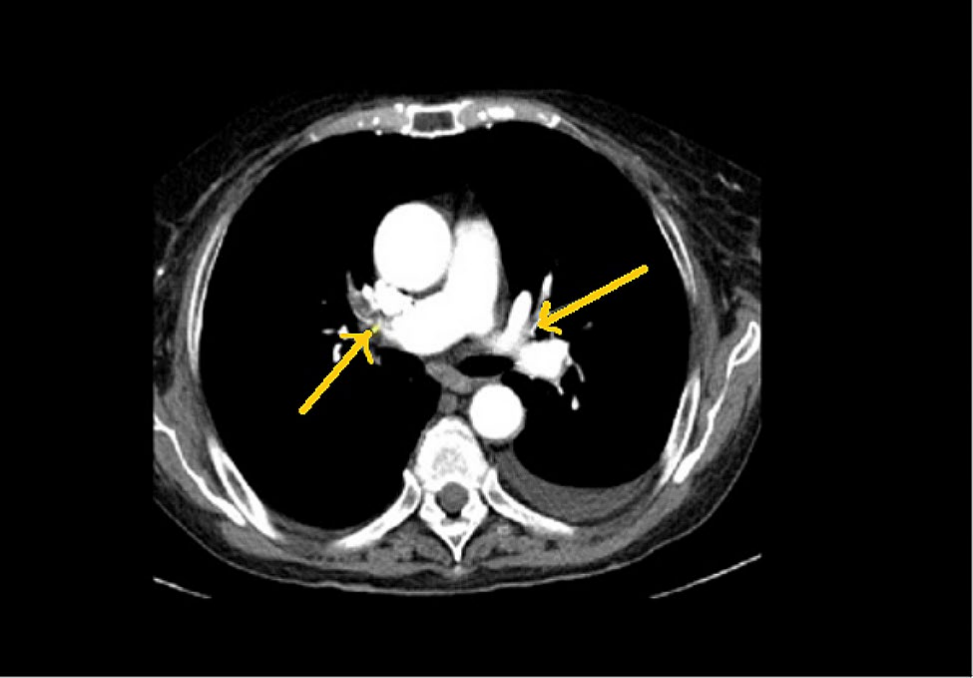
Initial bilateral pulmonary thrombembolism. Angio-CT examination, axial section, reveals thrombus in pulmonary artery bilateral (yellow arrows)
